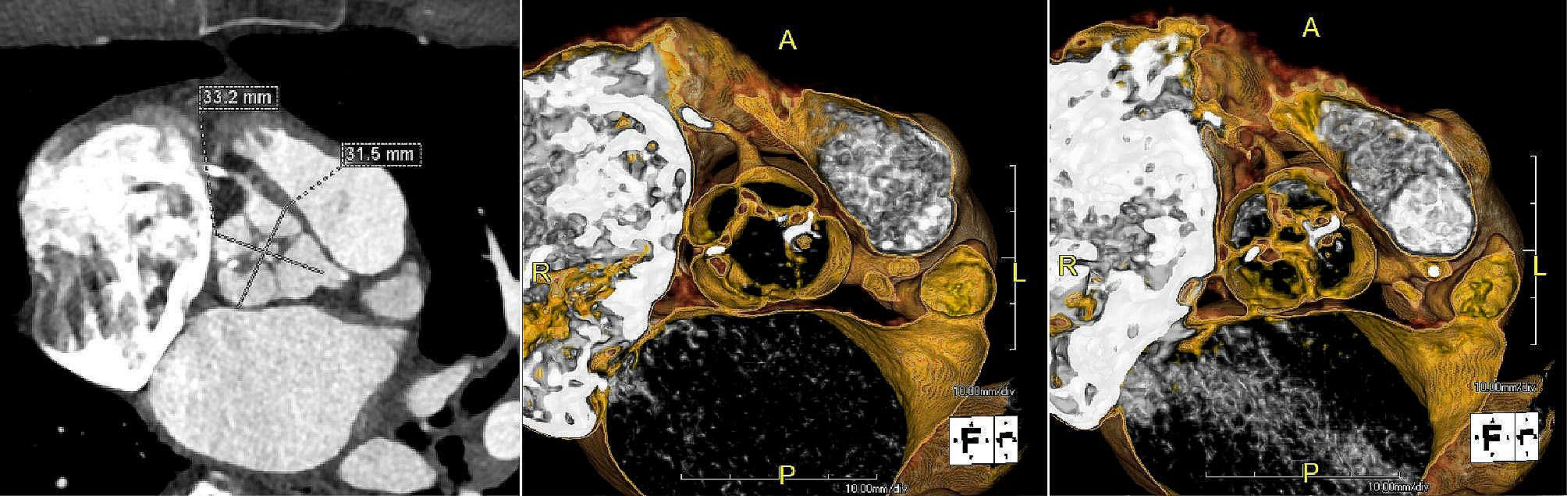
Pre-operative Computed Tomography of Quadricuspid Aortic Valve – Case A. (Left) 2-dimensional slice depicting quadricuspid aortic valve *en face*. The annular diameter was measured at 32 × 33 mm, and the cusps appeared equal in size. The origins of the left and right coronary arteries can be seen in their usual anatomic position. (Middle) 3-dimensional reconstruction of the valve in systole. Asymmetry of leaflet mobility is apparent and is likely due to calcification/fibrosis that is causing physiologic stenosis. (Right) 3-dimensional reconstruction of the valve in diastole. Central area of non-coaptation can be appreciated, as well as the equal size of the leaflets
